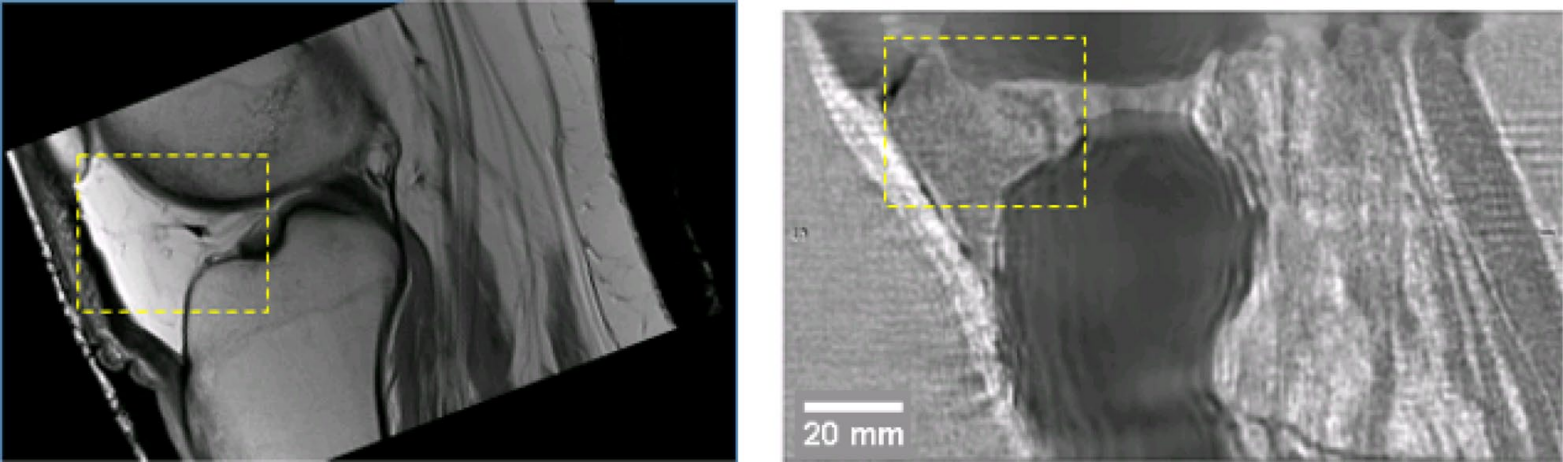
Comparison of the MR image (left) with the speed of sound image (right). The area enclosed in the yellow rectangle shows the Hoffa’s pad in both modalities. The correspondence is clear, even to the interface between the fat pad and the fat behind it, in the tibiofemoral space. The bone is clearly visible in the QT ultrasound image (QTUS). The high speed (light gray) muscle is seen in the SOS image and corresponds to the muscle showing up as a dark region on the MRI. The fat is light on the MRI, and dark (slow speed) on the QTUS image. The values from the QTUS image are quantitative correct to sub-mm resolution as shown in Tables 1 and 2.
